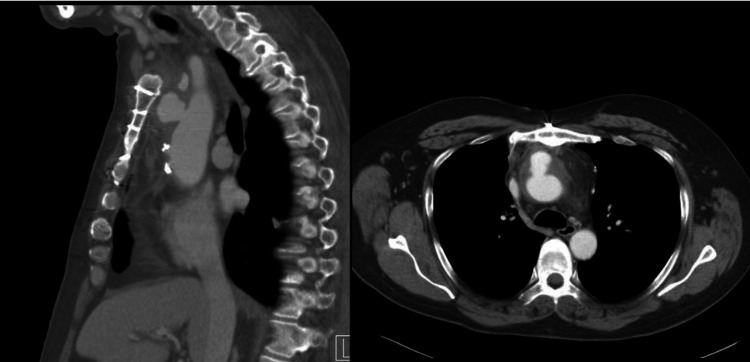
Chest computed tomography with contrast demonstrating a pseudoaneurysm in the anterior wall of the ascending aorta measuring 2.1 x 1.9 cm. The neck of the aneurysm measures 1.0 cm.
